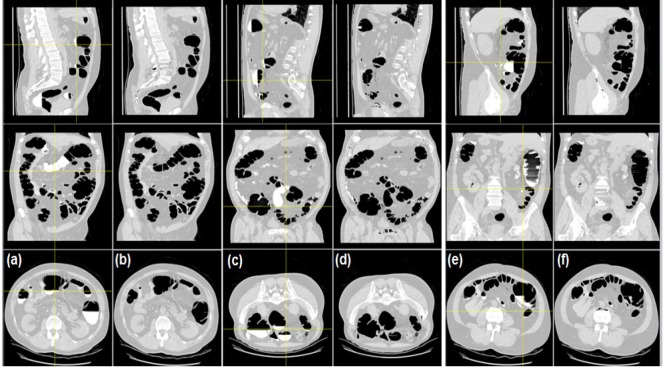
The MPR Planes of CT Volume with Left to Right (Axial), Anterior to Posterior and Axial Views (from the Top). Column a, b, c) Before cleansing and column a1, b1, c1) after cleaning of the colon. The colonic structures are preserved
